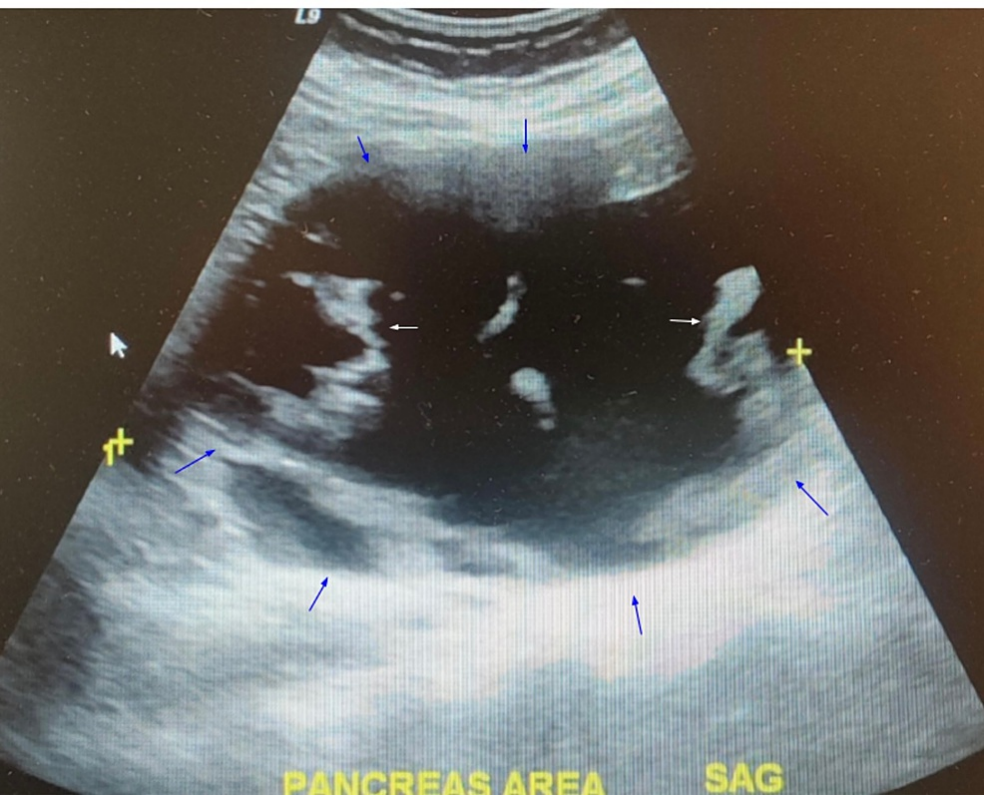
Ultrasound one week after percutaneous drainage. Blue arrows highlight the boundaries of the pancreatic cyst.
